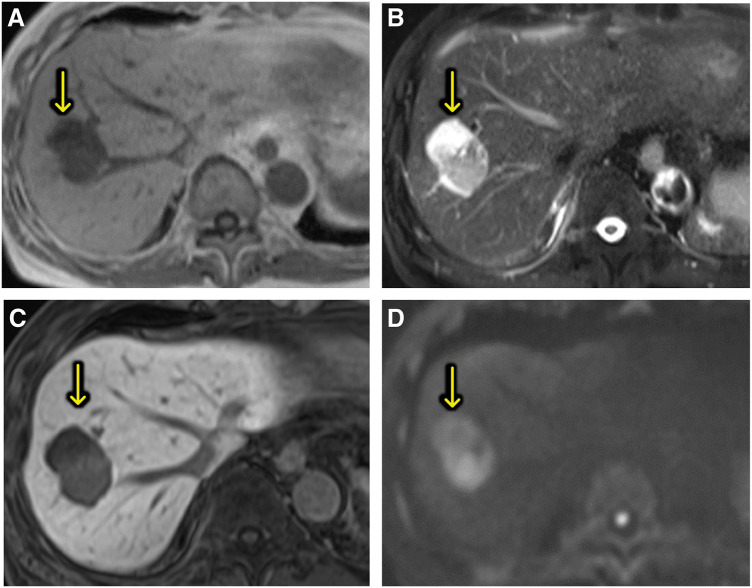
Magnetic resonance imaging findings of liver tumor. (A) T1-weighted imaging revealed a hypointense tumor (B) T2-weighted imaging showed relatively high signal intensity. (C) In the hepatobiliary phase, the tumor exhibited hypointensity. (D) Diffusion-weighted imaging demonstrated hyperintensity of the tumor.
